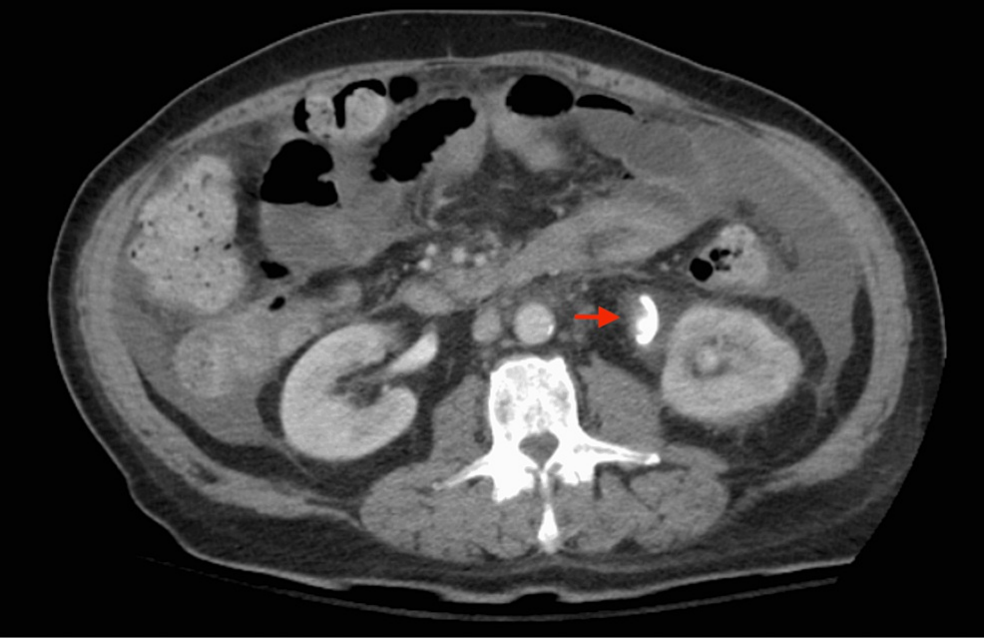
Computed tomography angiography on admission. Imaging revealed intravenous contrast in the distal portion of the ileal conduit raising concern for active extravasation.
